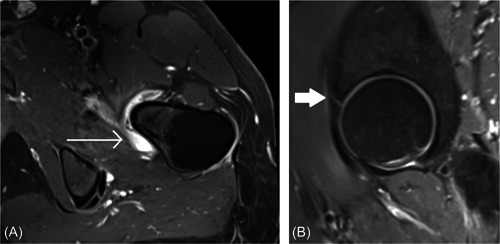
Hip osteoarthritis MRI score features. (A) Axial T1‐weighted fat‐suppressed sequence demonstrating grade 2 synovitis (thin arrow). (B) A sagittal proton density‐weighted fat‐suppressed sequence demonstrating a grade 2 labral tear (thick arrow). MRI, magnetic resonance imaging.
